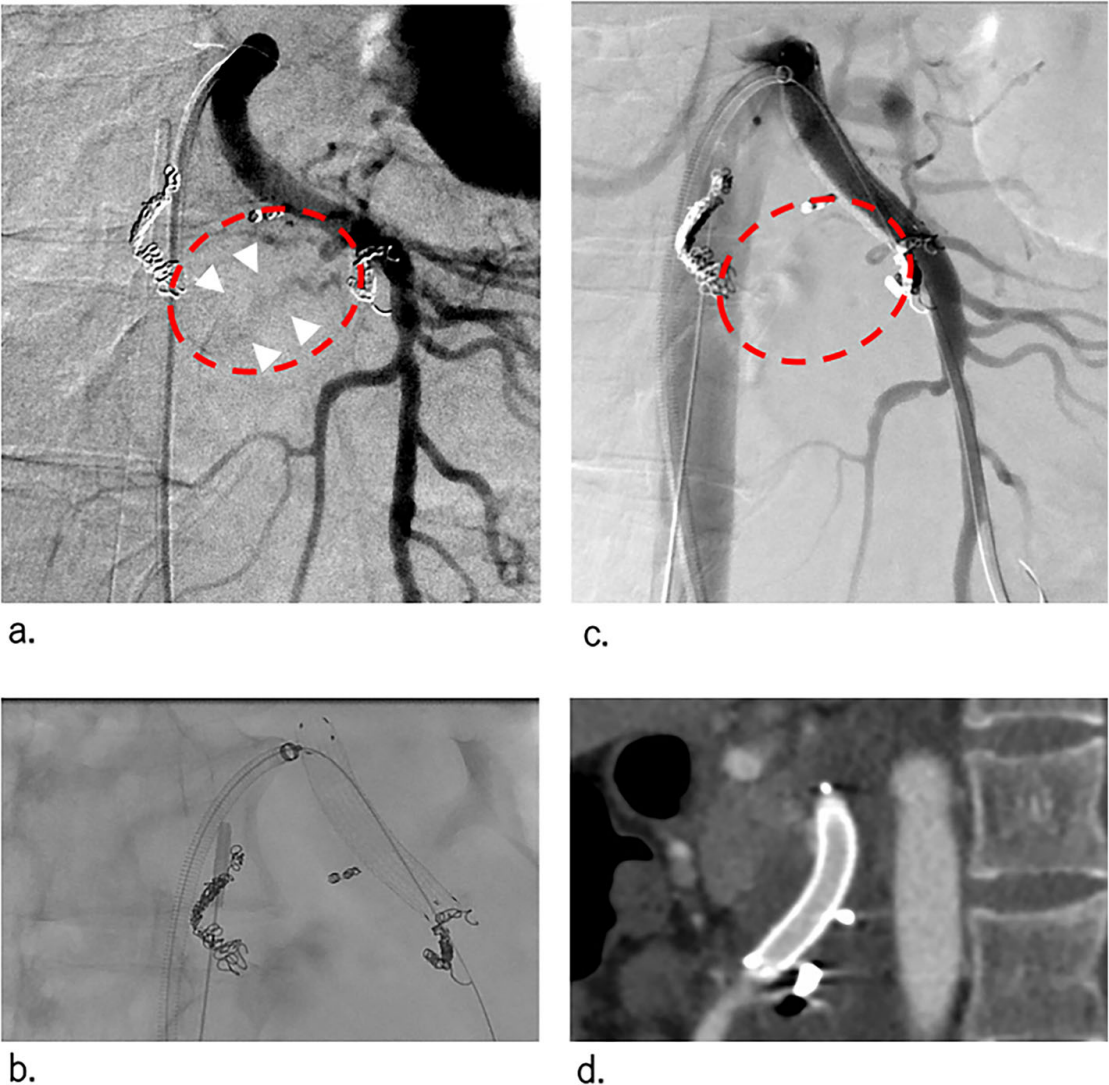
SMA angiography at stent-graft placement and postoperative computed tomography (CT) (a) SMA angiogram, obtained after TAE, showing continued extravasation (white arrowhead in the red circle) without a definite causal artery. b Digital angiogram obtained after stent-graft placement in the SMA trunk (Viabahn, 7 mm × 5 cm). c SMA angiogram, obtained after stent-graft placement, showing the complete disappearance of extravasation (red circle). d Contrast-enhanced CT image, obtained at day 28 after treatment, confirming good patency of the stent graft
